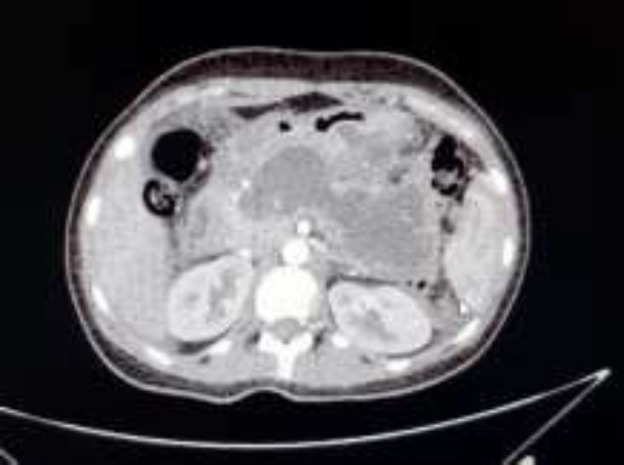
Abdominal CT scan: revealed edematous pancreas and enhancing loculi fluid accumulation around pancreas along with small amount of ascites fluid that all suggest acute pancreatitis
